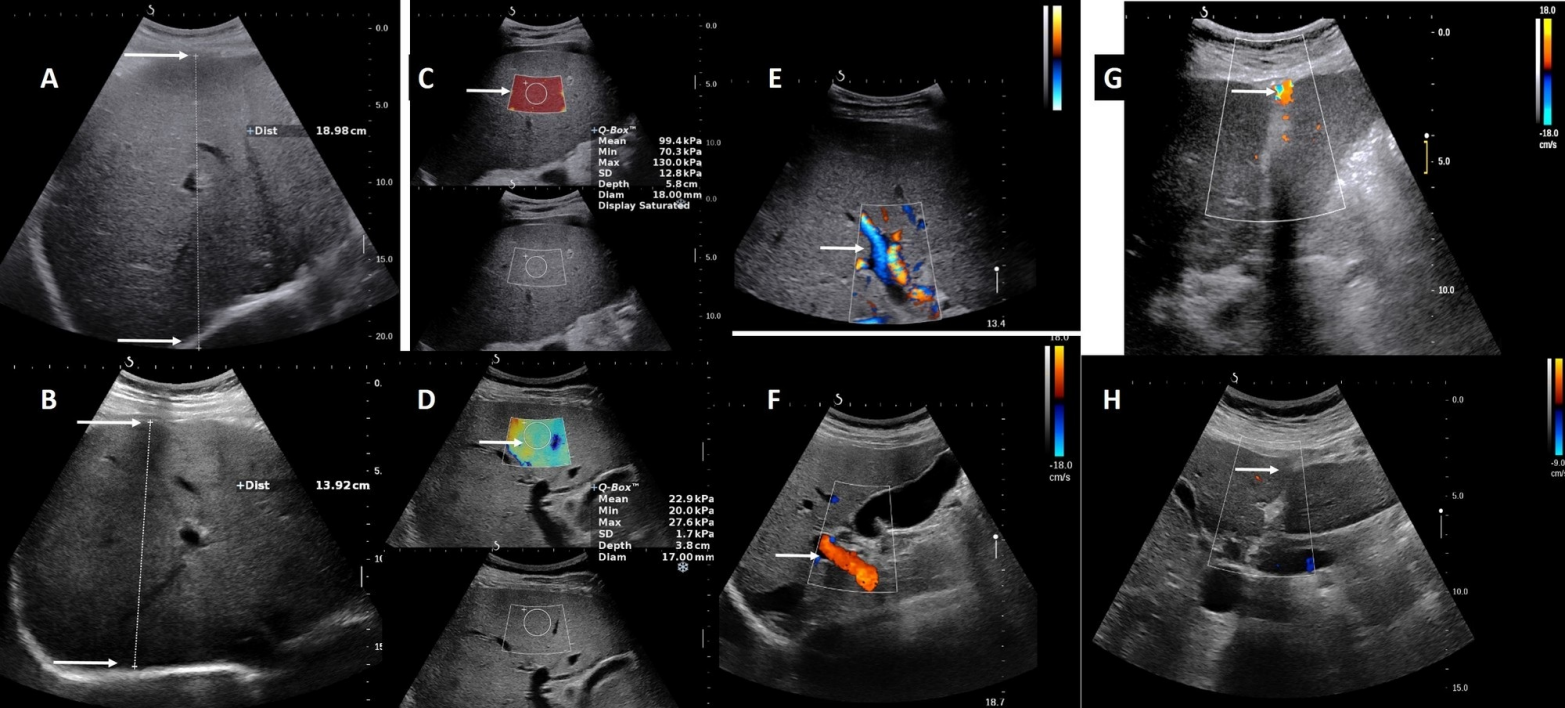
Ultrasound and 2D-SWE findings at presentation (October 2015, upper row) and 18 months after diagnosis (ongoing VGPR to chemotherapy; March 2017, lower row) The antero-posterior diameter of the right liver lobe measured at the mid-clavicular line was markedly enlarged on presentation (Panel A, arrow) and decreased on VGPR (Panel B, arrow). Similarly, liver stiffness by 2D-SWE was very high on presentation (Panel C, arrow) and decreased markedly on VGPR (Panel D, arrow). Intrahepatic portal blood flow was reversed (hepatofugal) on presentation (Panel E, arrow) and returned to normality (hepatopetal) on VGPR (Panel F, arrow). In addition patency of paraumbilical vein was noted on presentations (Panel G, arrow), and was no longer seen on VGPR (Panel H, arrow). 2D-SWE: 2-dimensional shear wave elastography; VGPR: very good partial response.
